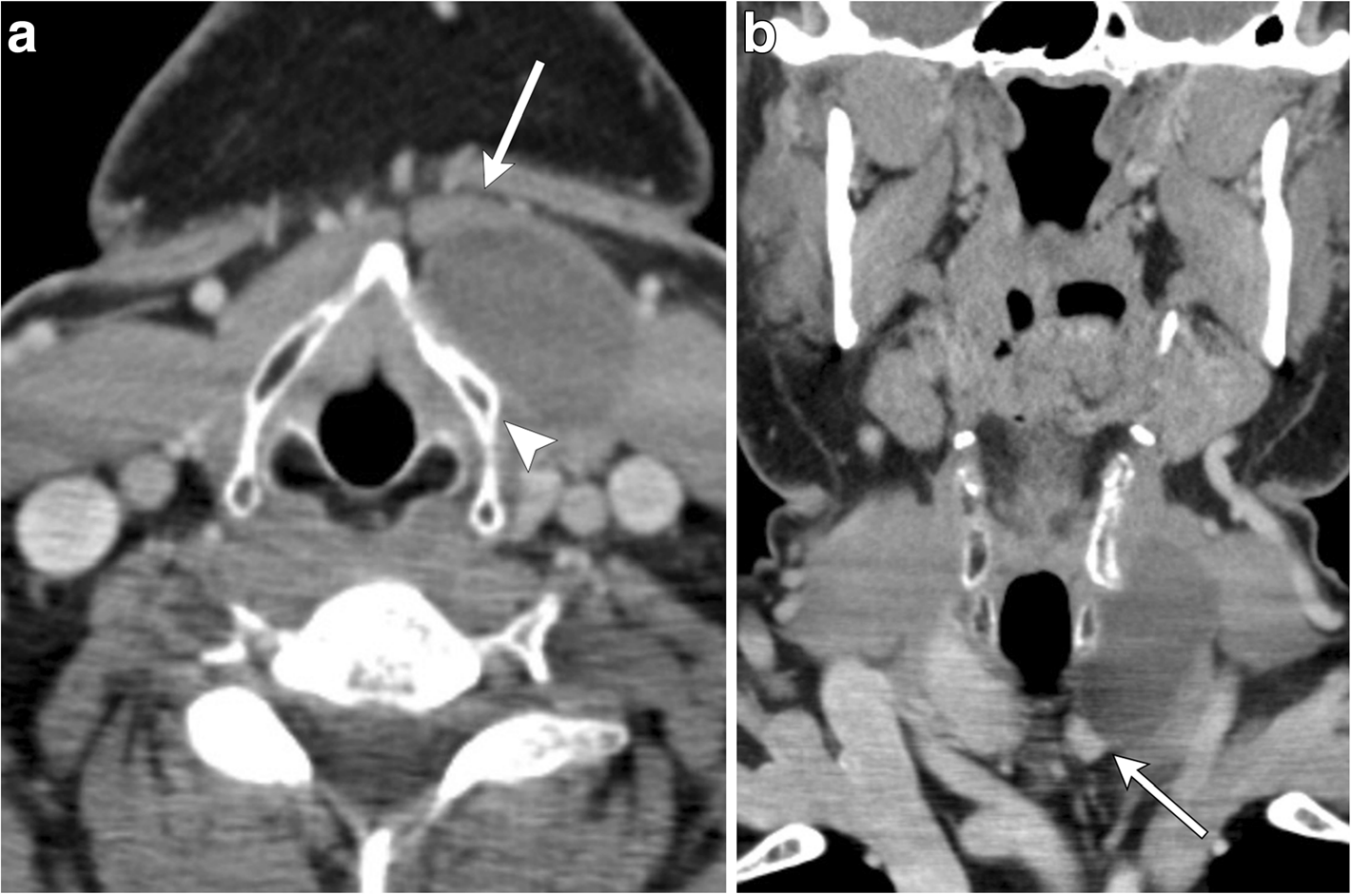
Paramidline thyroglossal duct cyst. a Axial contrast-enhanced CT images of the infrahyoid neck demonstrate a well-circumscribed homogenous low attenuation mass located between the thyroid cartilage (arrowhead) and strap muscle (arrow). b Coronal contrast-enhanced CT image shows the paramidline location and extension to upper pole of the left thyroid lobe (arrow)
